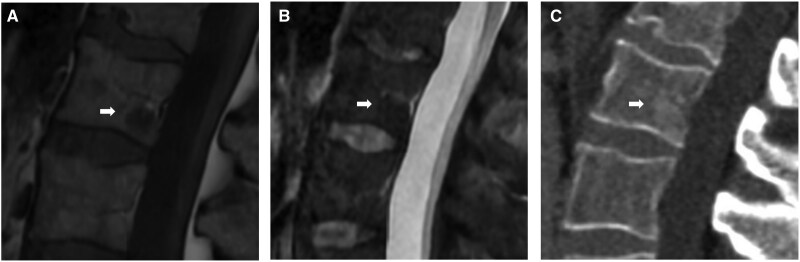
L2 vertebral body lesion (white arrow) in a 71-year-old male undergoing investigation for back pain and raised PSA. (A, B) Sagittal MRI sequences demonstrate focally reduced T1w (A) and STIR (B) signal. (C) Corresponding sagittal CT lumbar spine shows an ill-defined region of subtle sclerosis (C).
